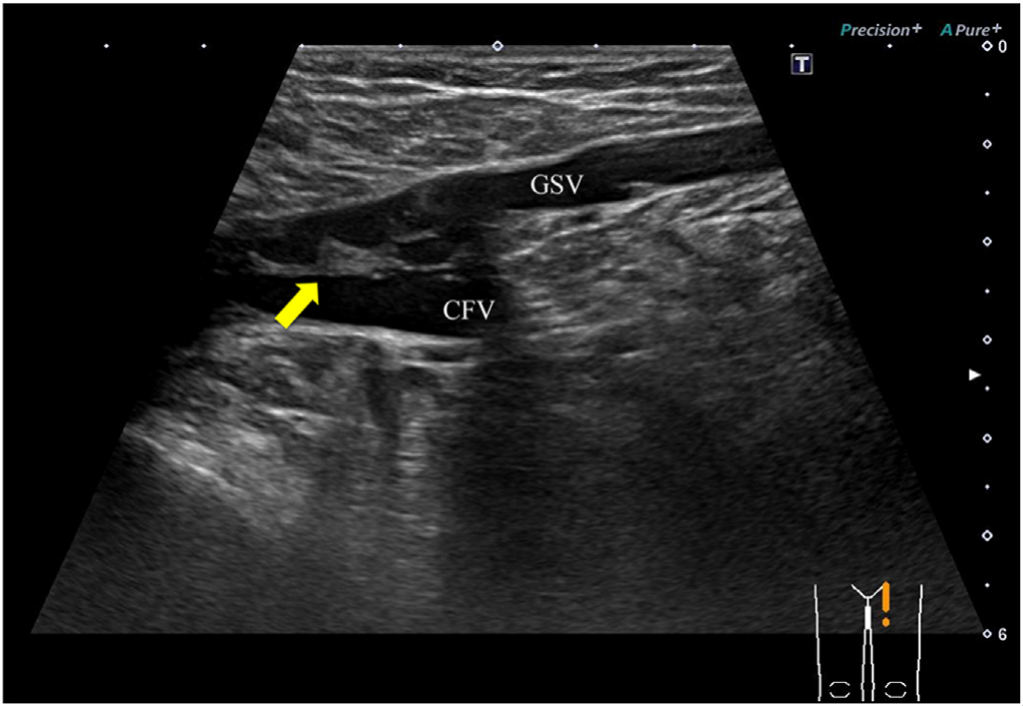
Ultrasound image showing post-thrombotic trabeculae (*arrow*) in the common femoral vein (CFV) extending to the saphenofemoral junction (SFJ). *GSV,* Great saphenous vein.
